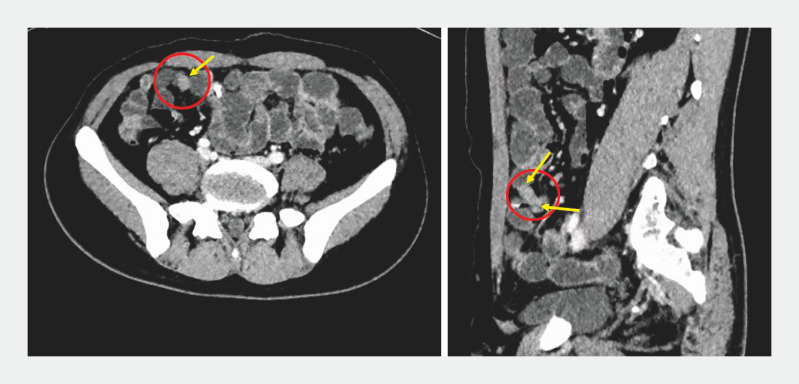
Computed tomography images showed a blind tube-like structure near the right lower abdomen and ileum.
